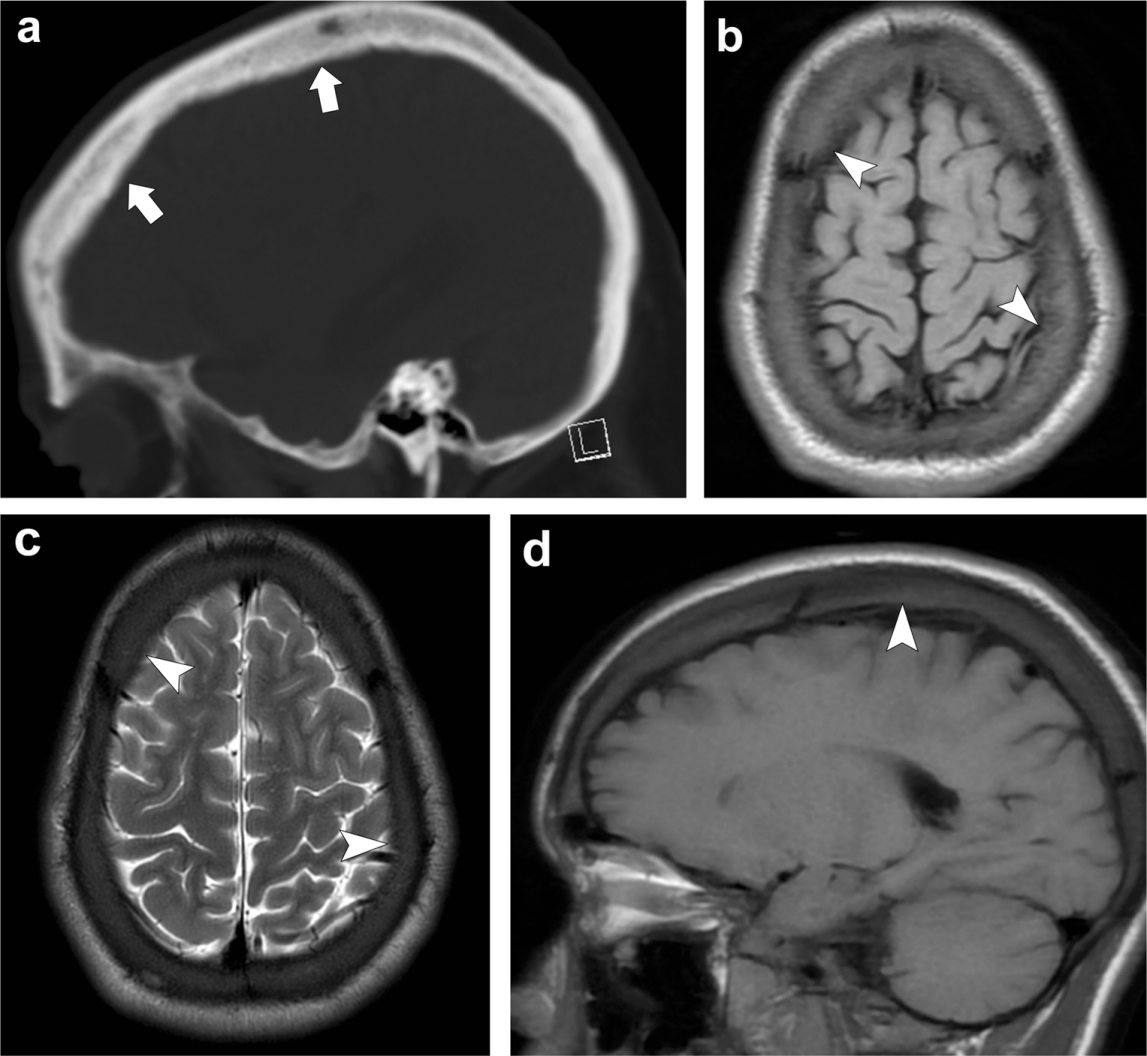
Chronic anaemia. Sagittal head CT (a) depicts widening of the diploic space (*thick arrows*). Axial T1-weighted (b), axial T2-weighted (c) and sagittal T1-weighted (d) images portray widening of the diploic space with generalised T1 and T2 marrow hypointensity (*arrowheads*) consistent with red marrow hyperplasia in this patient with sickle-cell anaemia
